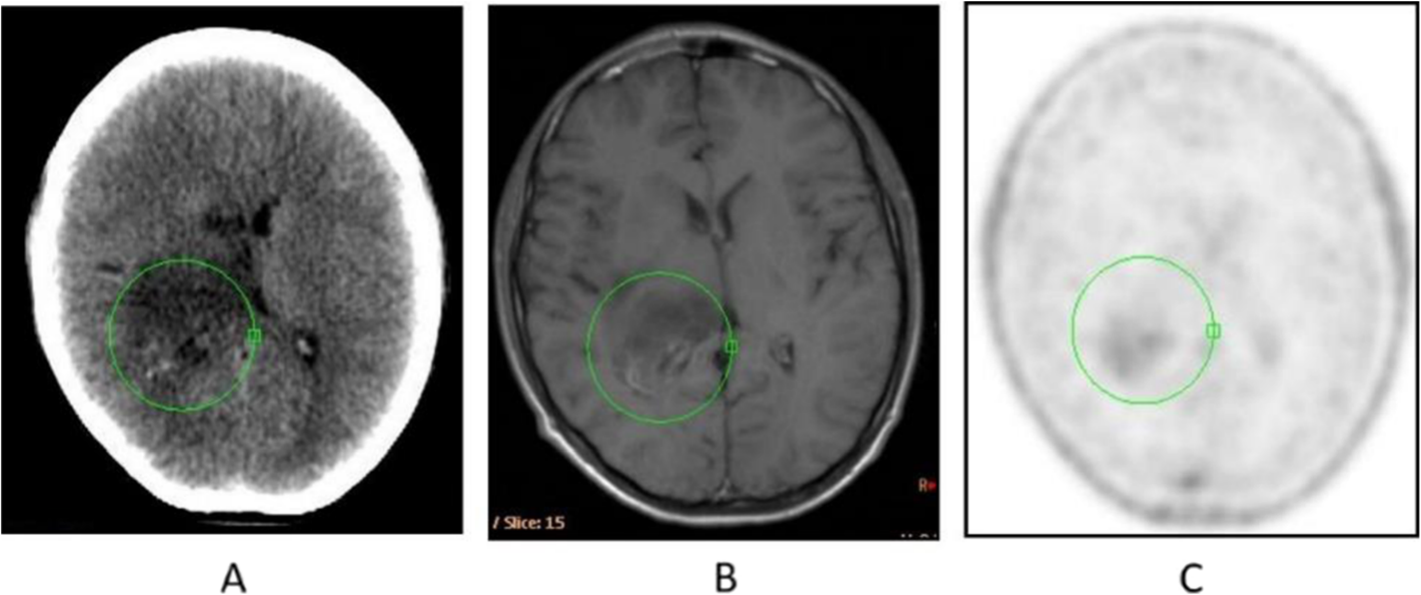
^18^F-FET, evaluation of a brain lesion. *Clinical history*: 46 y.o. man with seizure. CT (a) and MRI (b) shows an infiltrating lesion in the right parietal region (a). *PET/CT findings*: faint FET uptake in PET (c). Surgery confirmed a low-grade glioma
